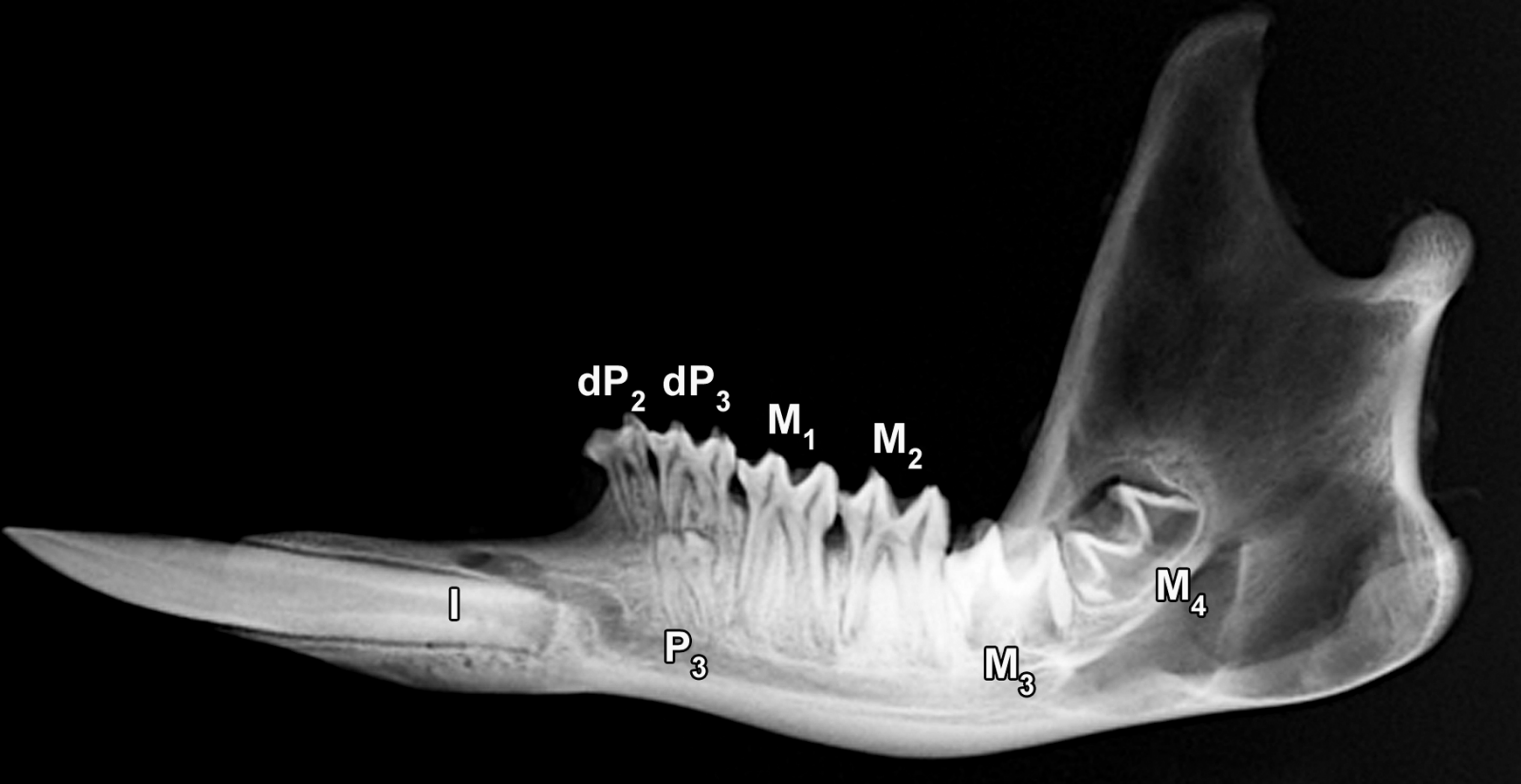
Lateral radiograph of the right mandible of an eastern grey kangaroo, *Macropus giganteus* (individual No.1153 (female), estimated age 2.3 yr) from the high-fluoride area. The radiograph shows the single large procumbent lower incisor (I) and the (erupted and unerupted) cheek teeth (premolars dP_2_, dP_3_ and P_3_, and molars M_1_ to M_4_). Of the cheek teeth, dP_2_, dP_3_, M_1_ and M_2_ are in function.
